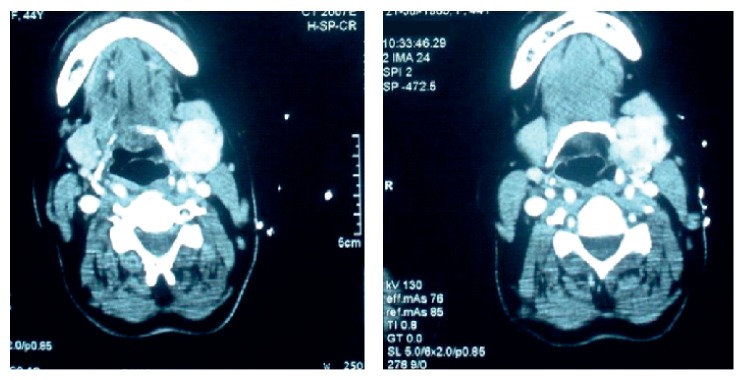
Postcontrast computed tomography findings; a well-circumscribed left submandibular mass.
